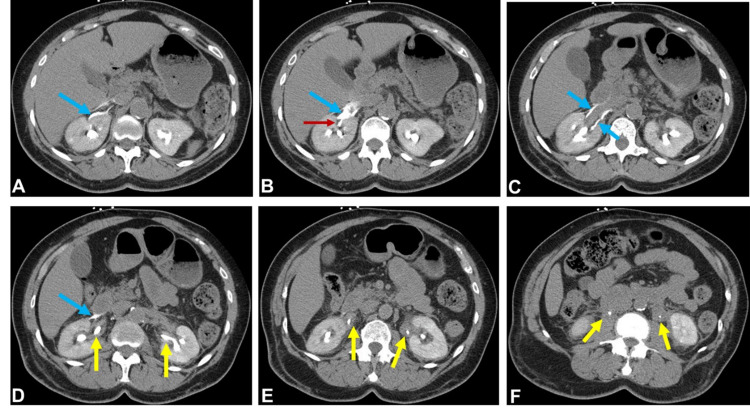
Axial contrast-enhanced CT of the abdomen obtained in the renal excretory phase with consecutive images through the kidneys (A-D) demonstrate accumulation of contrast outside of the collecting system and in the right perirenal space, also outlining the right renal vein (blue arrows). A focus of contrast contributing to this collection was seen arising from a right superior pole fornix (red arrow), compatible with forniceal rupture. Images obtained through the bilateral renal pelves (D), proximal ureters (E) and more caudal ureters (F) demonstrate prominent and high-density circumferential thickening of both renal pelves and ureters (yellow arrows).
